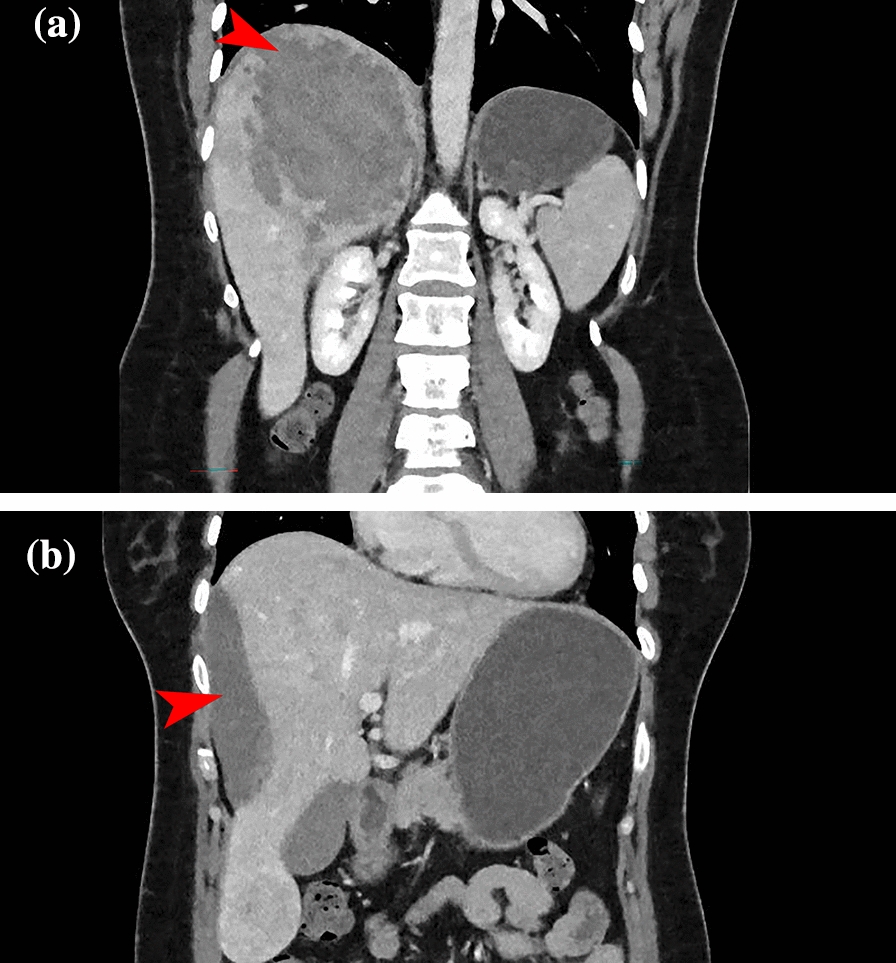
Initial abdominal computed tomography at presentation in the peripheral center, showing a large adenoma with post-bleeding status in segment 7 (A, marked with red arrow) and an extensive sub-capsular hematoma (B, marked with red arrow). No contrast extravasation is visible, indicating no active bleeding at the time of the computed tomography scan
